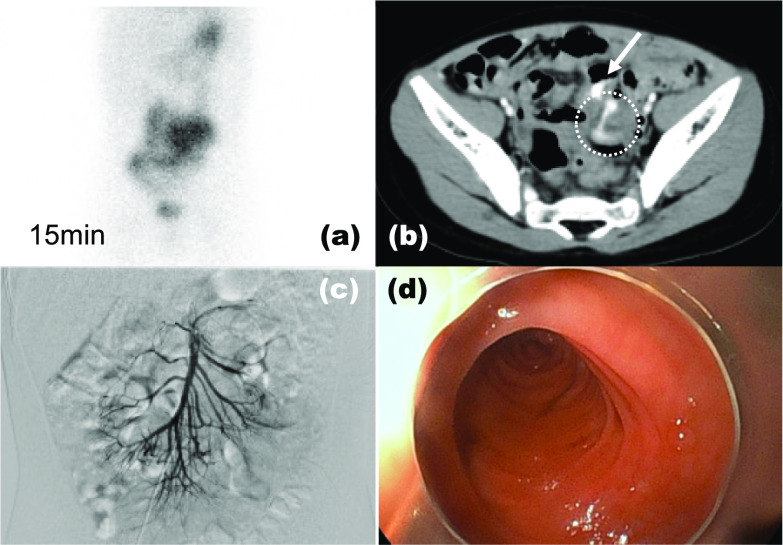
Preoperative images: a Tc-99m pertechnetate scintigraph. A part of the intestine is diffusely contrasted. b Enhanced CT showing extravasation into the small intestine (arrow) and the hypervascular lesion (dotted circle). c Arterial angiography indicating no remarkable extravasation. d Tran-sanal double-balloon enteroscopy showing no obvious abnormality on the mucosal side from the Bauhin valve up to 100 cm into the small intestine
